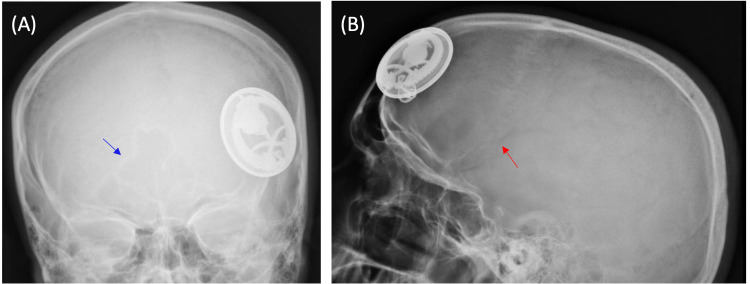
X-ray of the skull coronal view (A) and sagittal view (B) showing a metal foreign body on the left frontal bone. The blue arrow points to the frontal paranasal sinus, while the red arrow indicates the groove of the middle meningeal artery and its branches on the inner side of the temporal-parietal bone.
